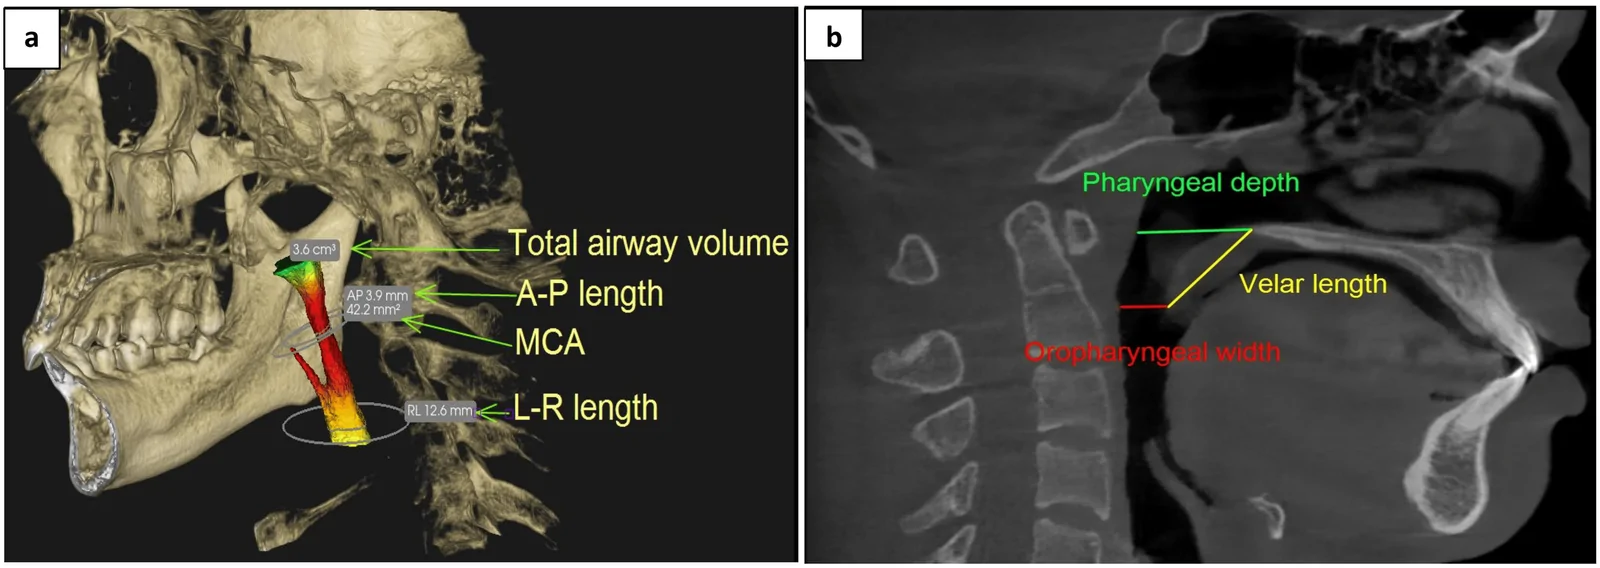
Cone beam computed tomography-based measurement protocol (a) Software interface (CS 9600 Airway Analysis Tool, version 3.10.39) showing automated computation of airway volume, minimum cross-sectional area, and minimum anteroposterior and transverse distances. (b) Linear parameters measured on the mid-sagittal section, including velar length, pharyngeal depth, and oropharyngeal airway width.
